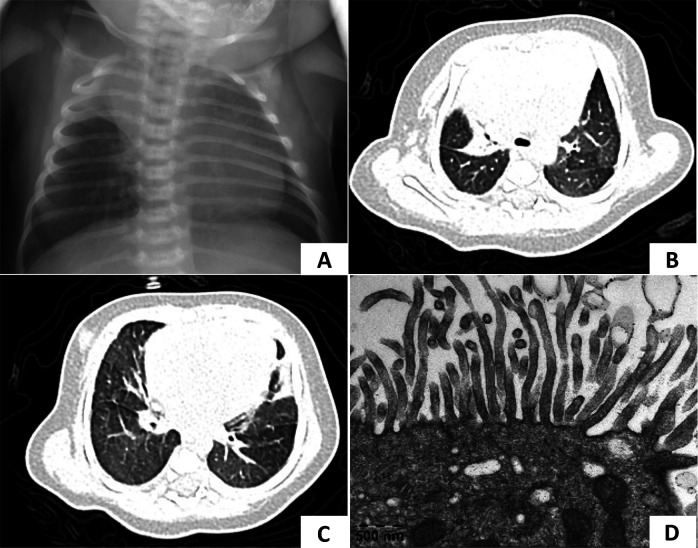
Chest radiography and TEM of case 2. (A) Chest x-ray showed atelectasis of the right upper lobe at 1 week after birth. (B,C) At 1 month of age, a lung CT scan showed a decreased volume of the right upper lobe and patchy opacities in both lungs which demonstrated pneumonia with right upper lobe atelectasis. (D) TEM showed microvilli with few cilia.
